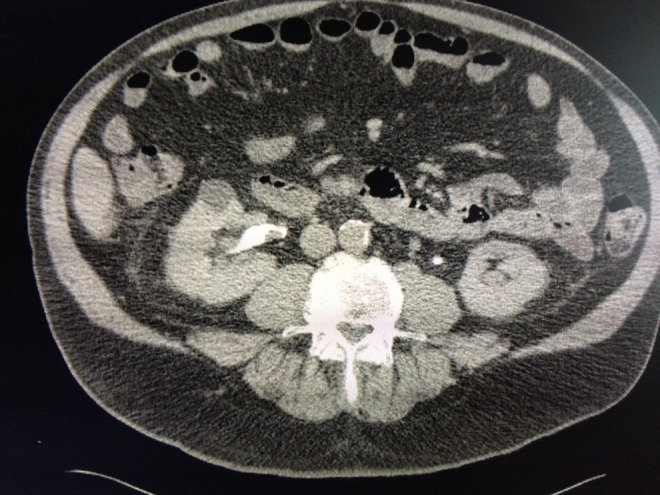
Axial view of CT urogram demonstrates a 1.5 cm filling defect in the right renal pelvis.
